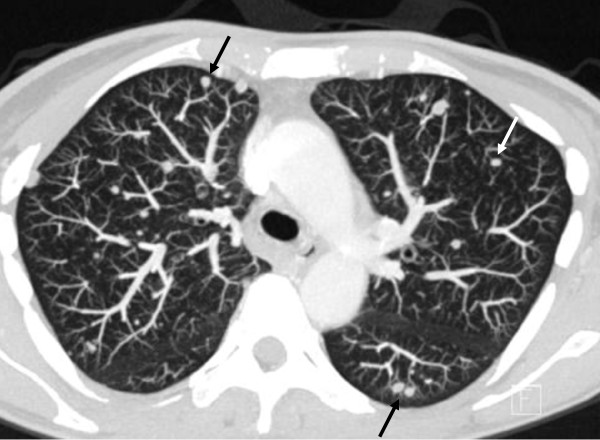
Computed tomography scan of the thorax after five cycles of chemotherapy showing multiple metastatic lesions (black and white arrows) corresponding to pulmonary metastasis.
